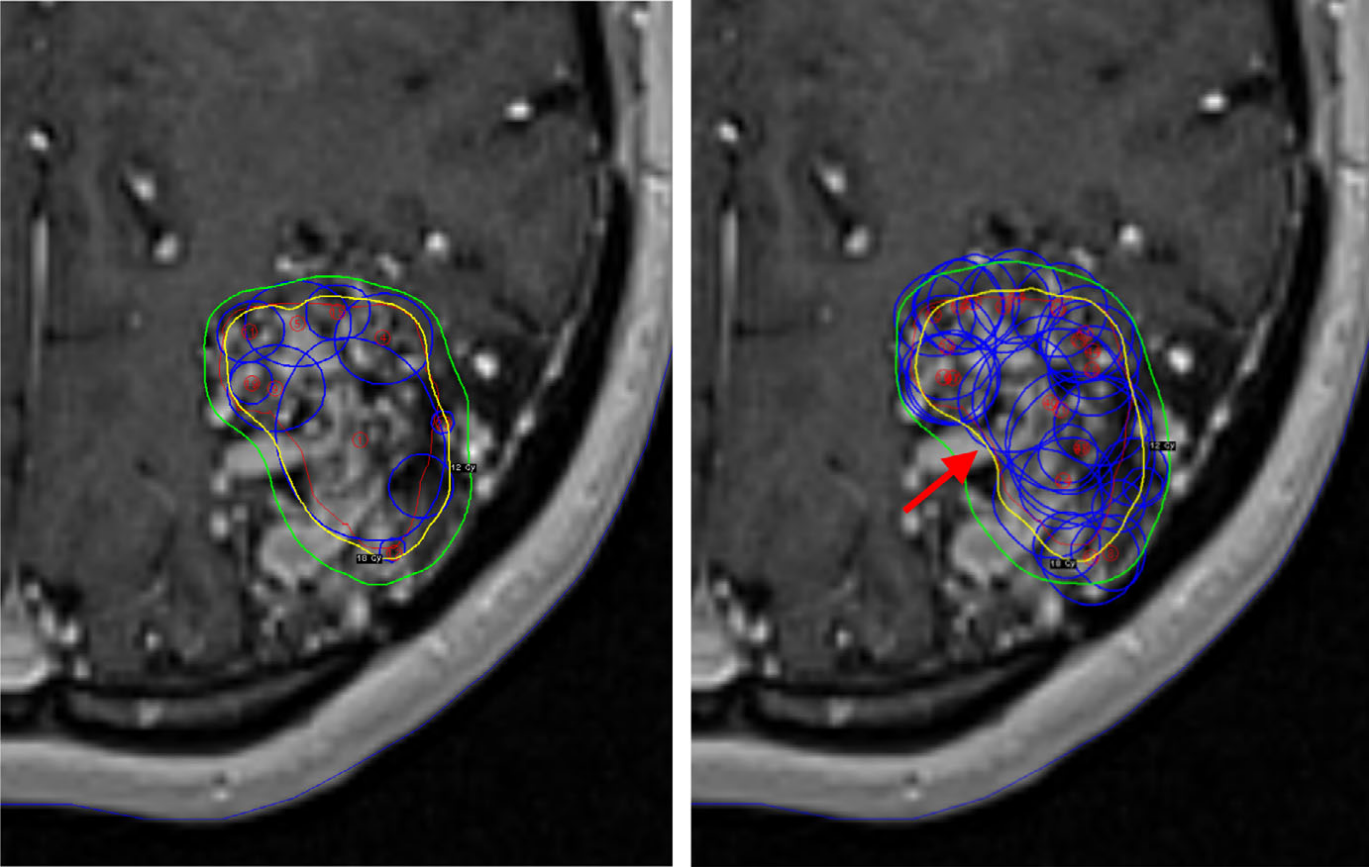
Transaxial T1 weighted magnetic resonance imaging slices with the manual (left) and inverse (right) plans for case number 6. The blue circles denote the isocentre size and position. The 18 Gy (prescription dose) and 12 Gy isodose lines are shown in yellow and green respectively. The improved concavity of the prescription isodose (red arrow) on the medial edge of target is shown on the inverse plan.
